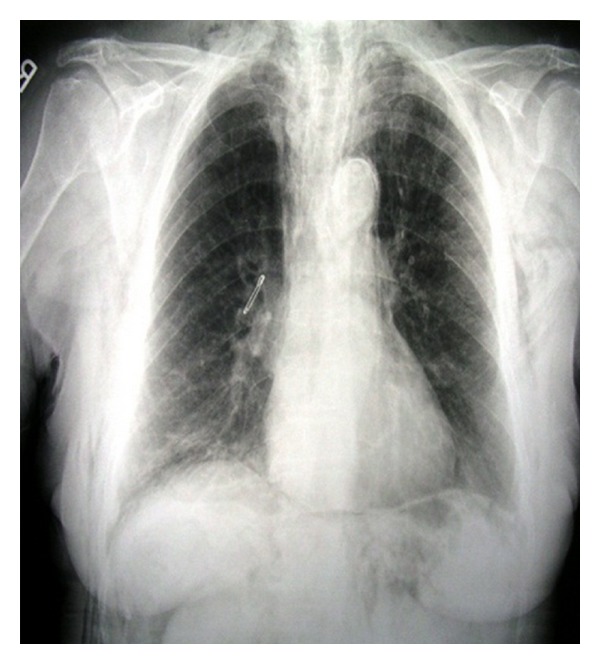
Chest X-ray demonstrating diffuse subcutaneous emphysema, pneumomediastinum, and subdiaphragmatic free air.
